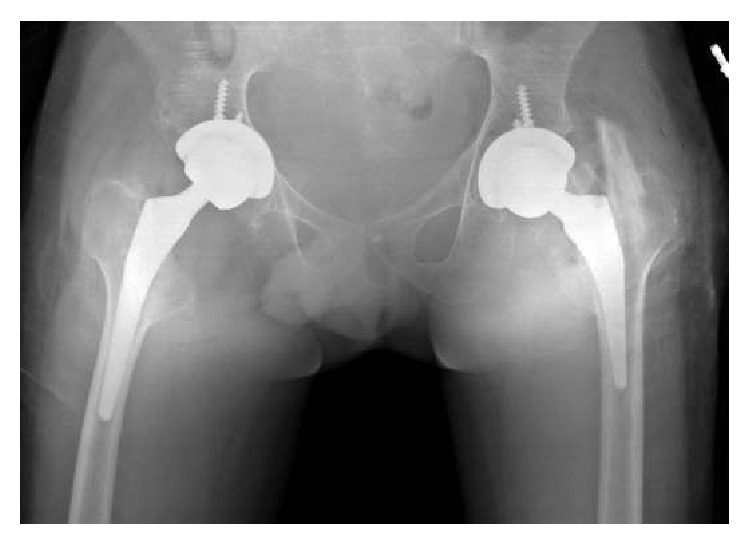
Anteroposterior both-hip radiograph depicting bilateral cemented THA at last follow-up.
